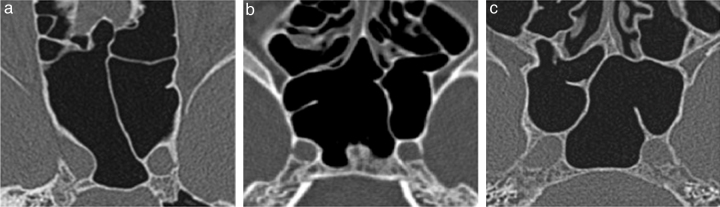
Septation type definition and its relationship with the internal carotid artery protuberance (ICAp). Axial reformatted images obtained from CT data scanning. (a) Intersphenoid septation in the left ICAp. (b) Intersphenoid septation in the left ICAp and an accessory septation in the left sinus wall. (c) Intersphenoid septation in the right ICAp and an accessory septation in the left ICAp.
